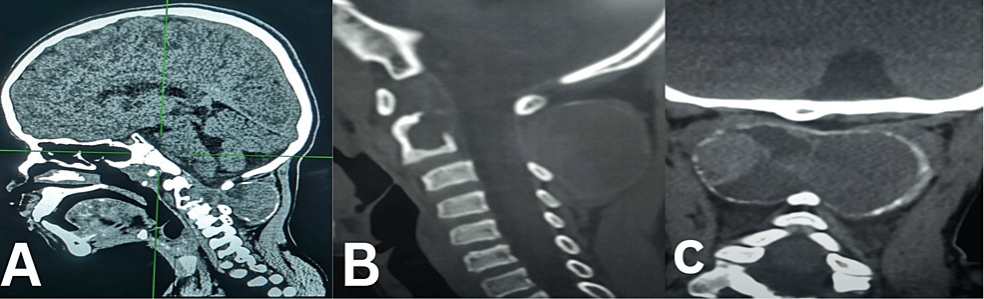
Neck CT scan. (A) Sagittal view with soft tissue window. (B) Sagittal CT bone window with C2 posterior elements, multiloculate bony cyst with osteoclastic changes, and thinned cortices, mainly posterior elements. (C) CT scan coronal view with the same findings as in panel C.
